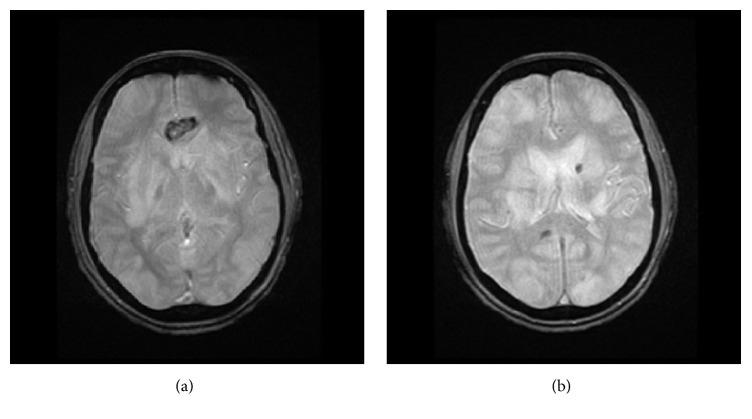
MRI Brain-Axial gradient echo-weighted images demonstrate susceptibility effect in the genu and splenium of the corpus collosum as well as the left frontal periventricular white matter compatible with multifocal hemorrhage.
